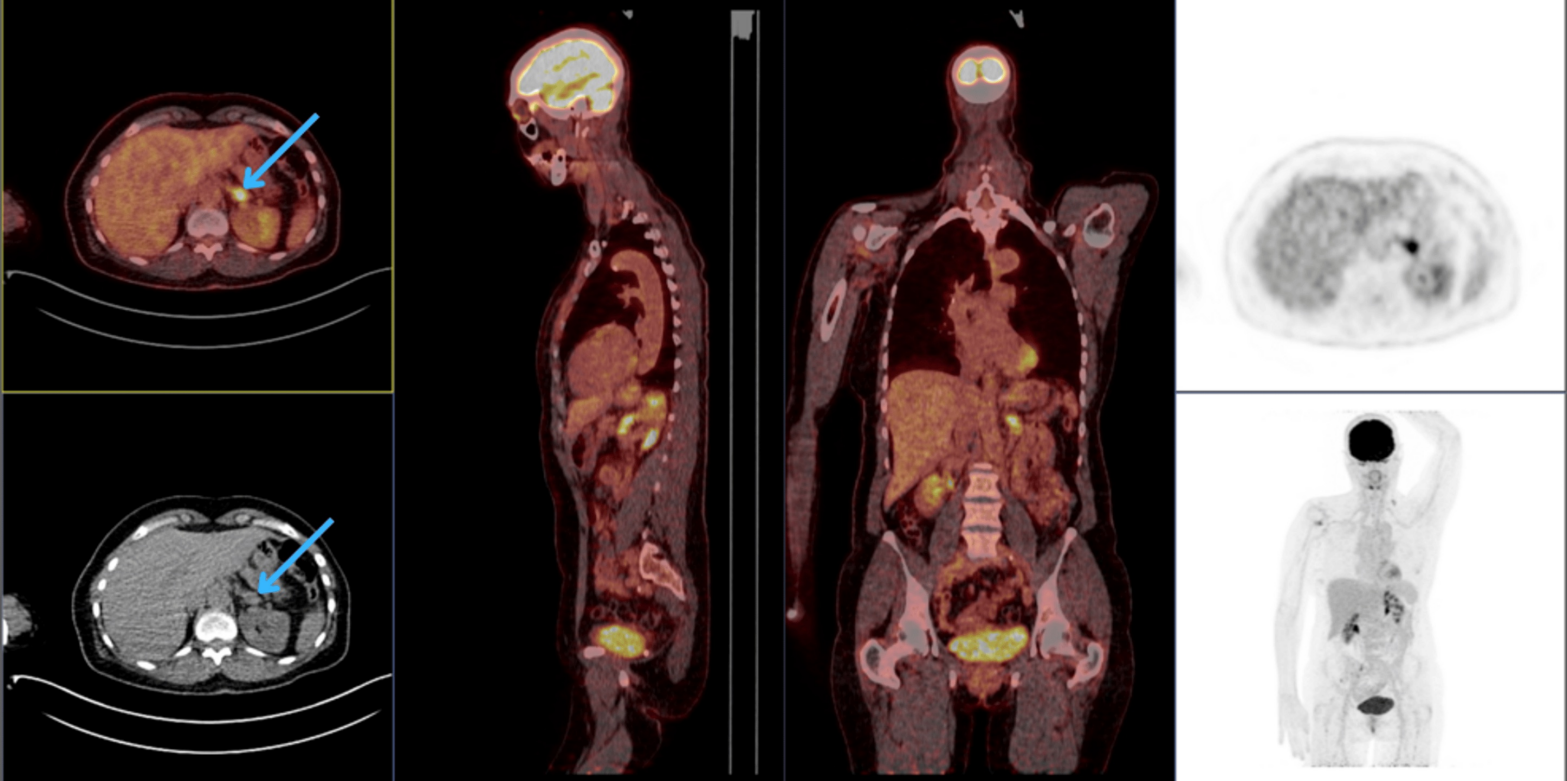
The PET-CT scan showing left adrenal metastasis (blue arrows) PET-CT: Positron emission tomography-computed tomography
